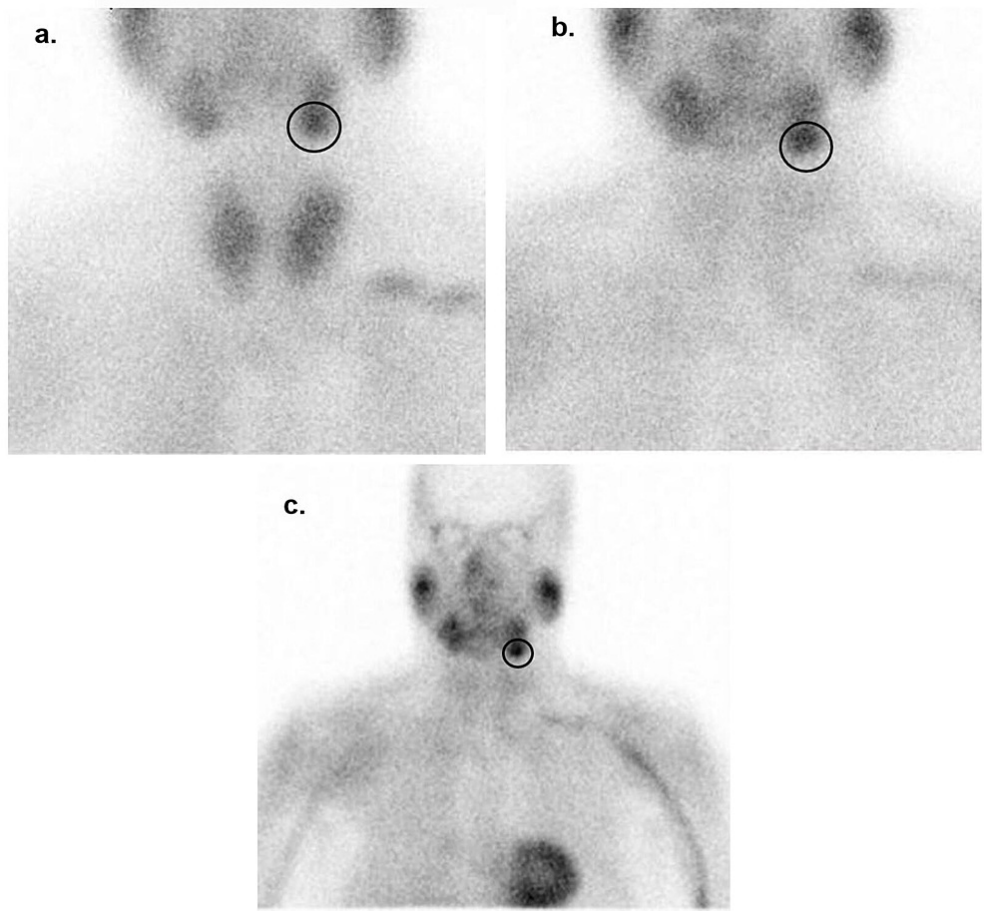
Technetium-99m sestamibi scintigraphy After administration of the radiopharmaceutical, (a) 10 minutes; (b) and (c) three hours. Black circles – pronounced uptake on the inferior region of the left submandibular gland.
